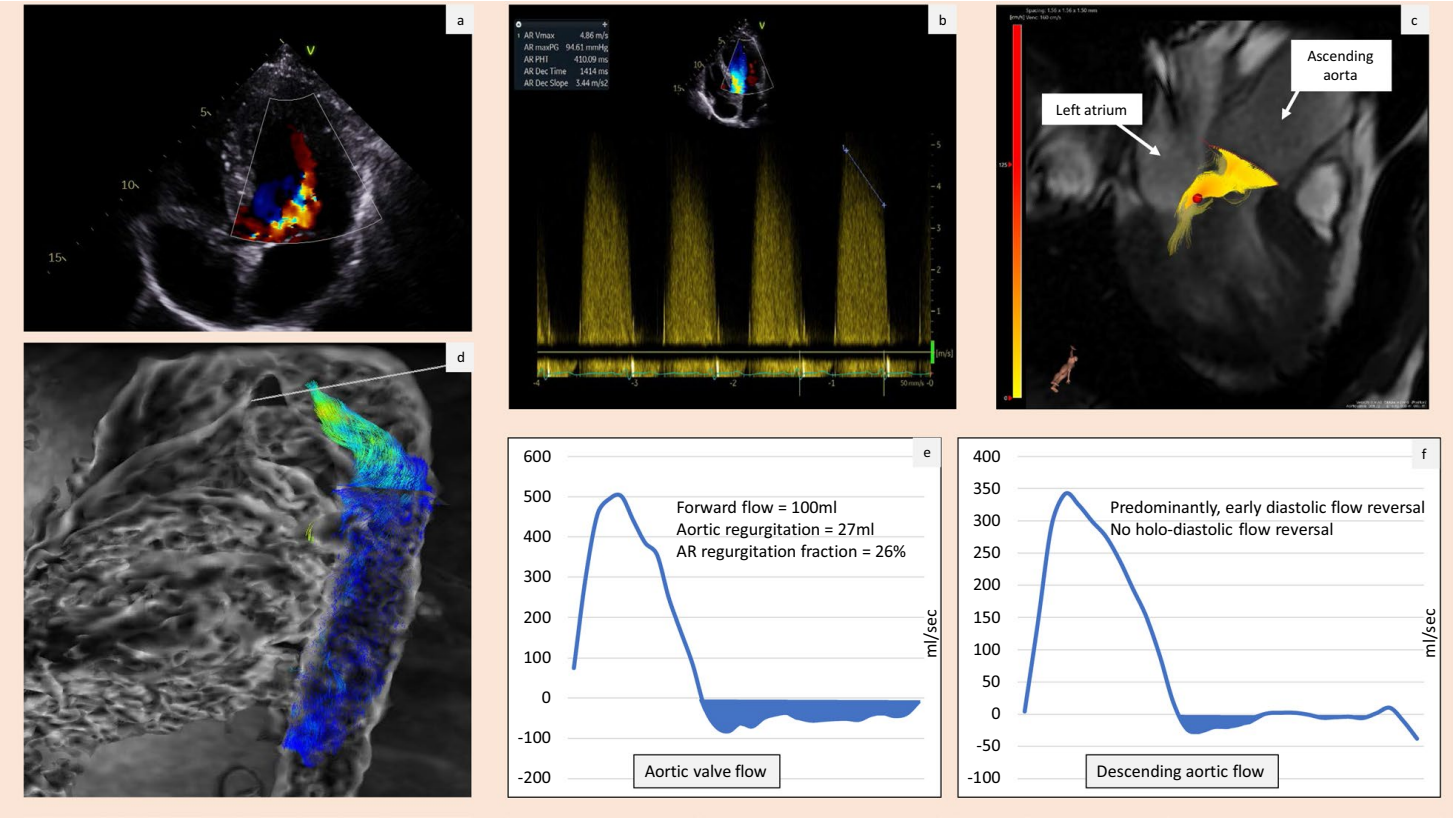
TTE and 4D Flow CMR assessment of moderate aortic regurgitation. a 4-chamber colour Doppler transthoracic echo (TTE) demonstrating aortic regurgitation jet and b TTE continuous wave Doppler demonstrating pressure half time consistent with moderate aortic regurgitation. c is a four-dimensional flow streamline visualisation of aortic regurgitation in left ventricular diastole. d is pathline visualization of flow reversal in the early diastole in the descending aorta. e is a reformatted c at aortic valve level flow quantification. f is the reformatted plane at the descending aorta level flow quantification
